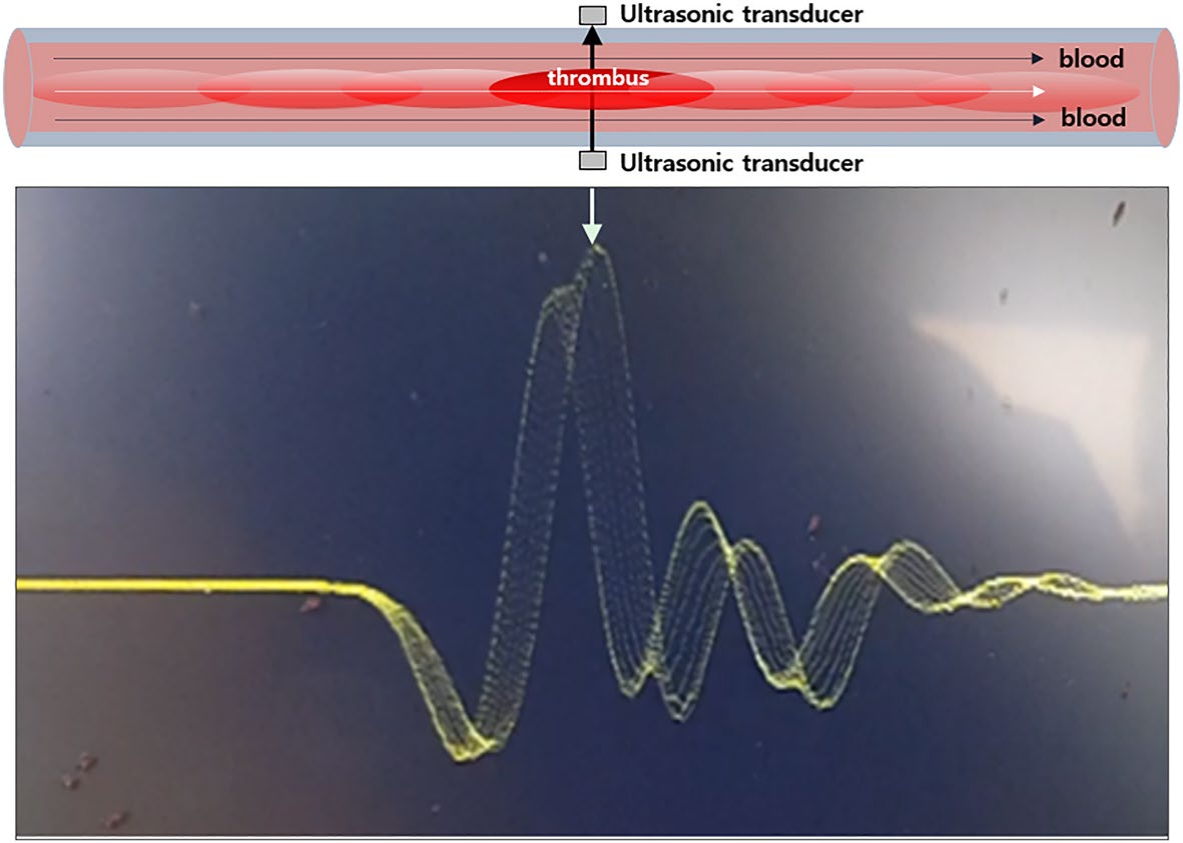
Thrombus pass through ultrasonic sensors. Thrombus flowing through the bloodline across the ultrasonic sensors. While the thrombus passes through the ultrasonic sensor, it induces multiple signal changes depending on the stages.
